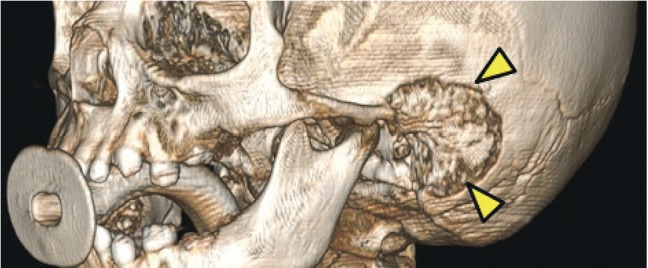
Cortical erosion of the temporal bone (yellow arrows) in a CT scan. 3D view.
